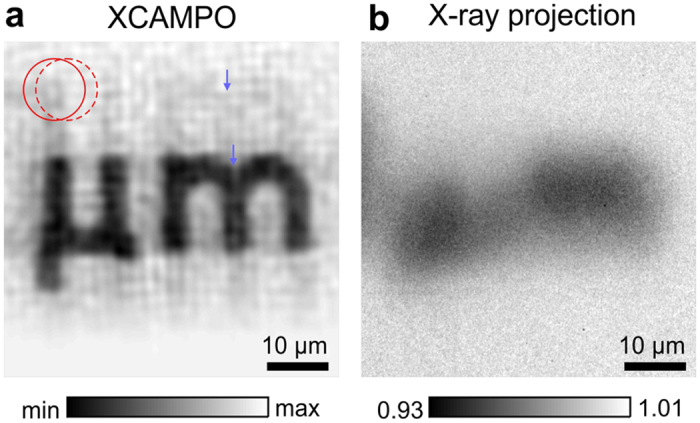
A reconstruction of a larger 2D object from a grid-enhanced XCAMPO dataset is presented in (a). For comparison, (b) shows a standard X-ray projection image obtained with the optic but without the grid. The centres of the red circles indicate two successive scanning positions, and their diameters reflect the focal spot size. The arrows indicate corresponding points in the real image and in a weak “ghost” image.
